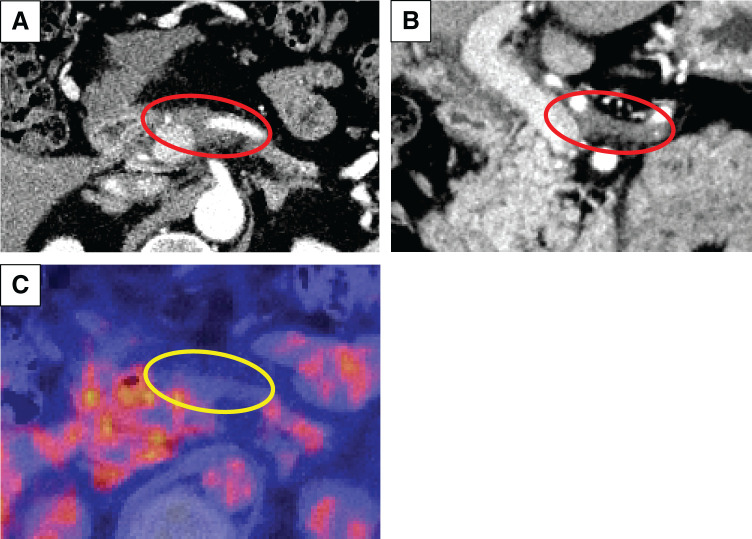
Contrast-enhanced PET-CT were performed just before surgery. (A, B) The red circle shows shrinkage of the primary tumor. (C) The yellow circle shows no uptake in the primary tumor.
